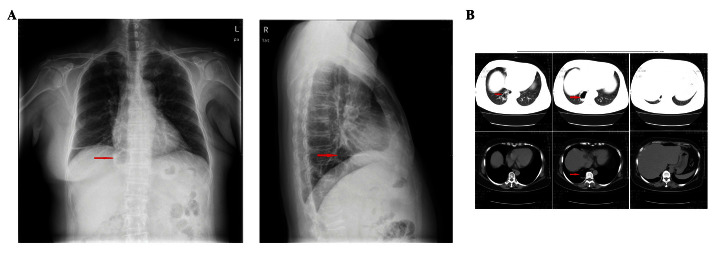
Images before lower lobectomy surgery. (A) The chest X-ray showed a striped high-density mass in the right lung above the diaphragmatic surface (arrow). (B) Chest computer tomography (CT) revealed a cystic low-density mass of ∼2.5×4.7 cm in the right lower lung field (arrow), as well as cystic bronchiectasis and pneumonia in the right lower lobe.
